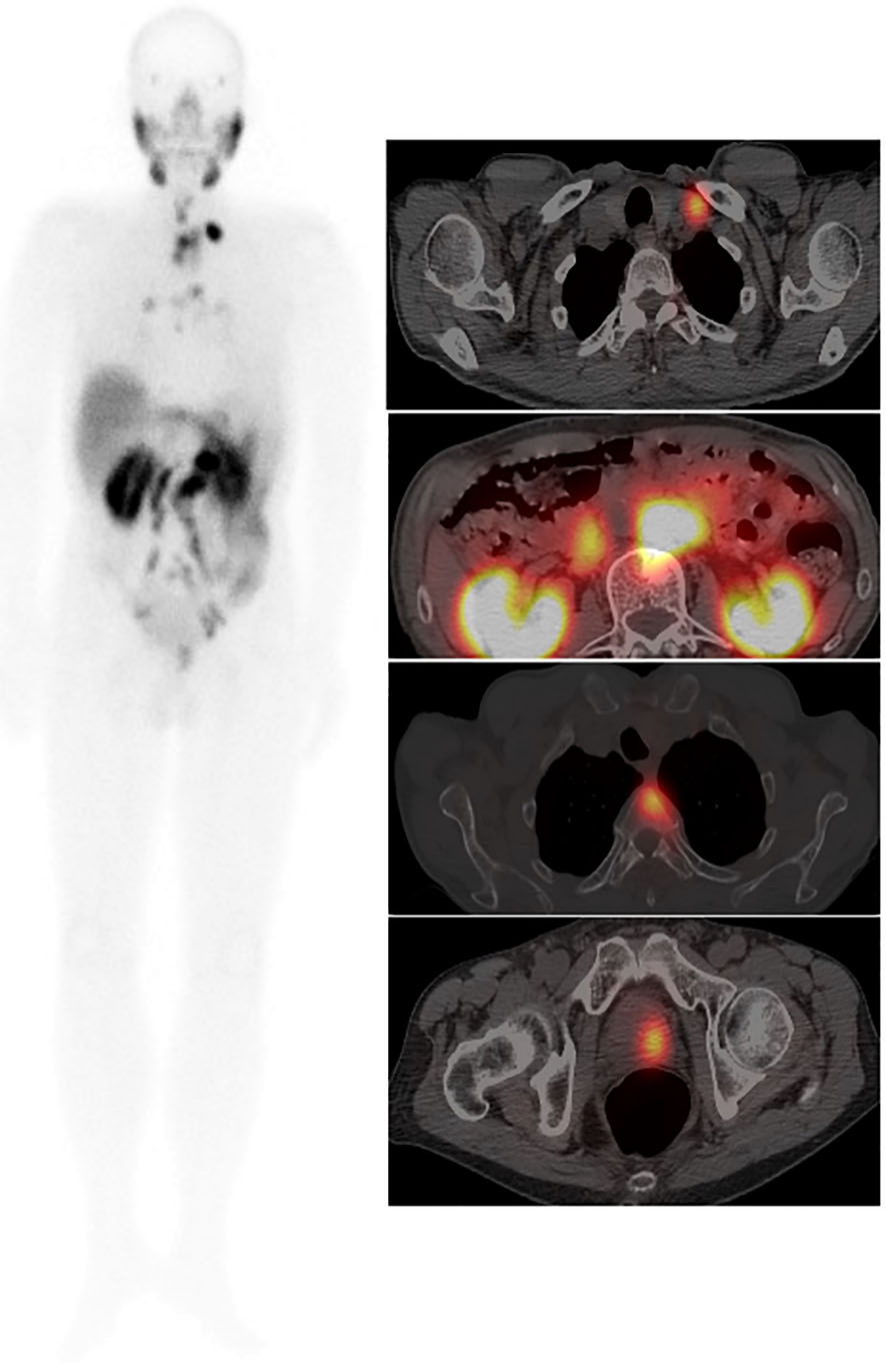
Male, 76 years, GS = 8 (4 + 4). tPSA 147.0 ng/ml. ^99m^Tc-HYNIC-PSMA whole-body scan planar (ANT) image (left) showed multiple PCa lesions. An axial fused image (right) showed primary tumors in the left prostate lobe, multiple lymph node metastases, and thoracic vertebral metastases.
